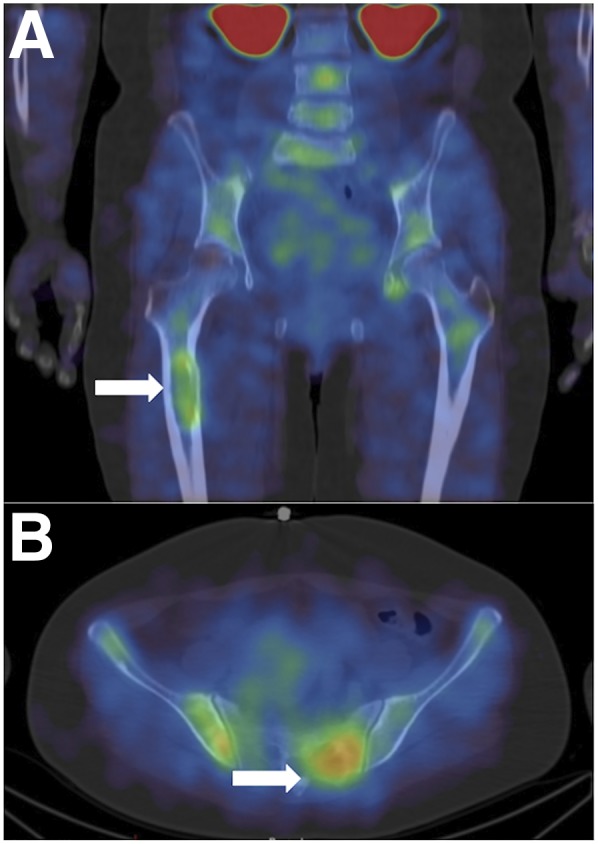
Coronal (A) and axial (B) ^99m^Tc-NM-01 SPECT/CT images of a patient with skeletal metastases (arrows) demonstrating PD-L1 expression.
